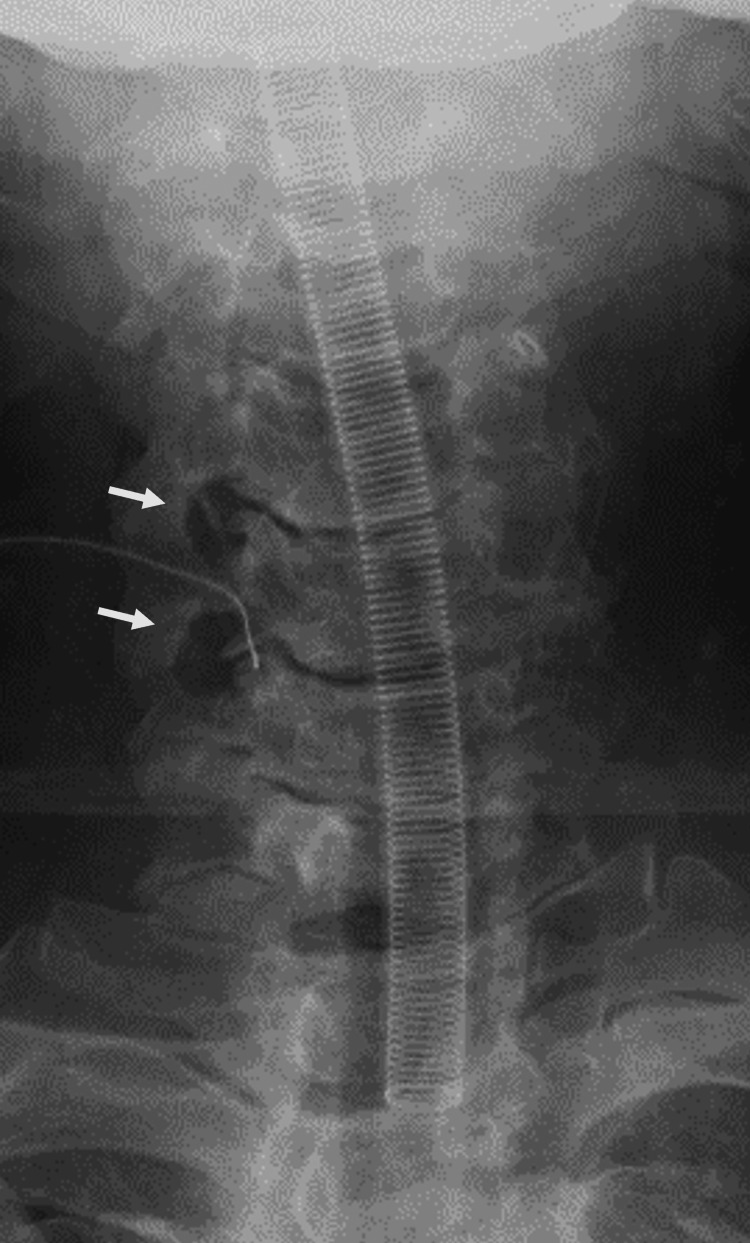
Postoperative anterior-posterior X-ray Posterior decompression is achieved beyond the Luschka joint. The arrows show the decompression sites
